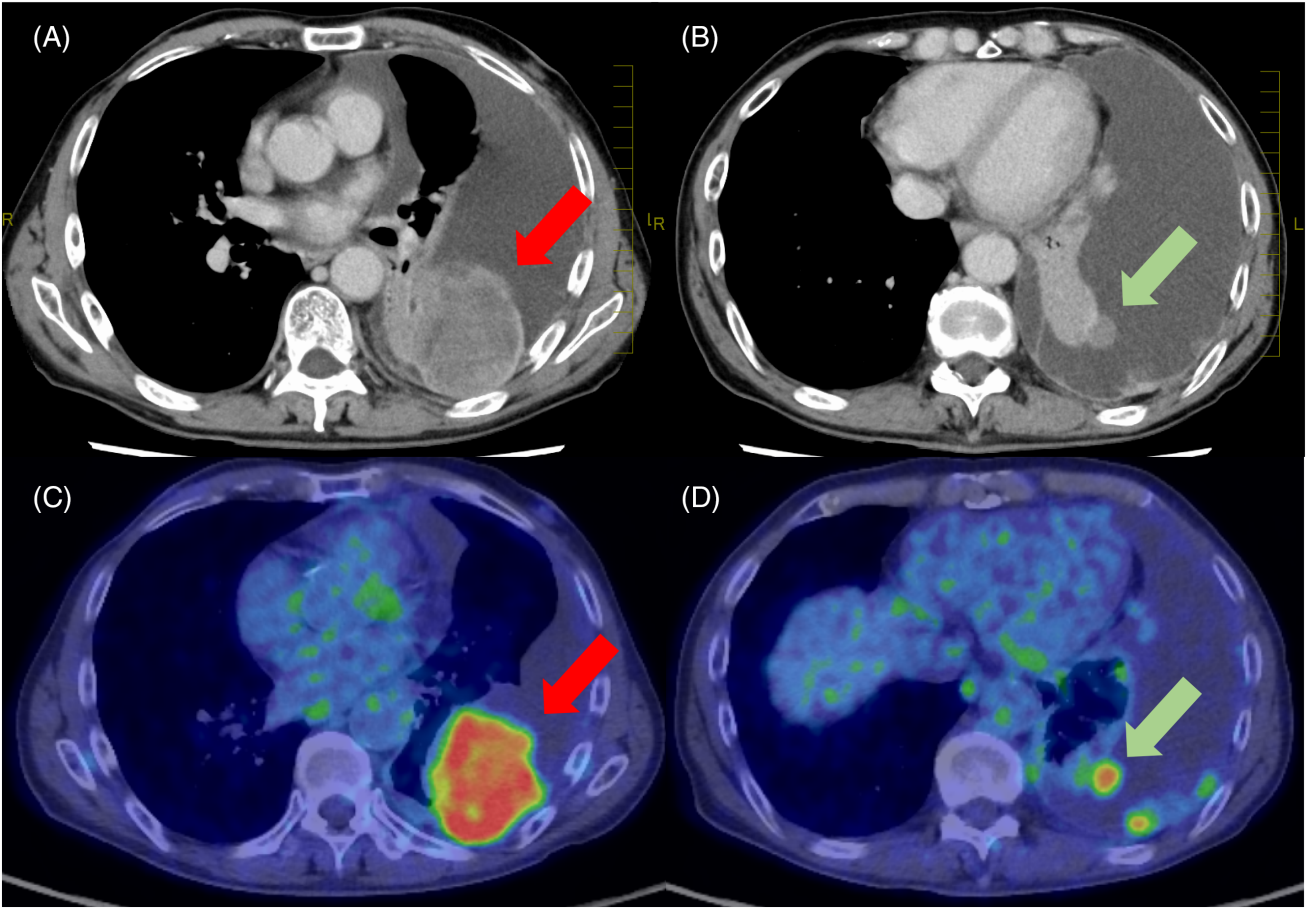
(A) Computed tomography (CT) image showing pleural effusion and a 65‐mm large mass (red arrow). (B) A 20‐mm convex nodule extending into the thoracic cavity from the visceral pleura (green arrow). (C, D) Positron emission tomography‐CT images showing fluorodeoxyglucose accumulation in each mass (red and green arrows)
